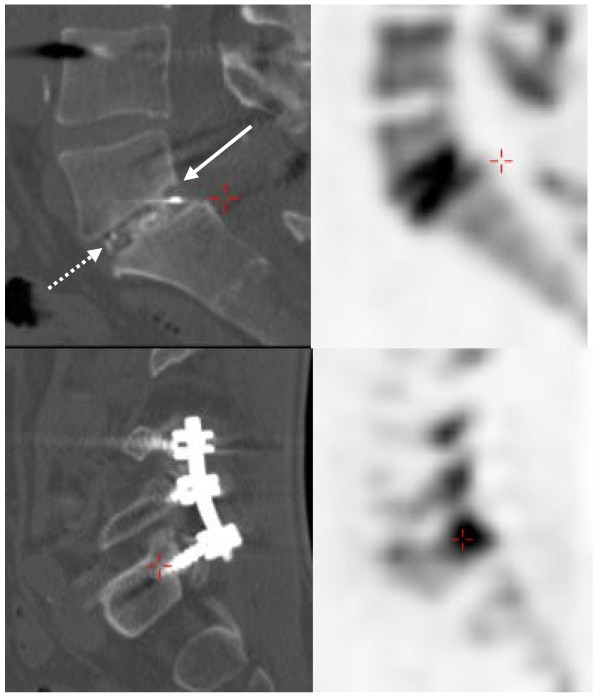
PET/CT after PLIF spondylodesis. Example of a patient, 7 months after PLIF for a lysis-olisthesis of L5-S1. Moderate subsidence on CT (upper left, closed arrow) and vertebral endplate activity on PET (upper right). Associated pedicle screw entrance point hyperactivity on PET (lower right, cross), possibly indicating continued bone stress post-surgery. The intervertebral area (dotted arrow) shows the bone fragments inside the cage and one of its posterior radiological markers.
